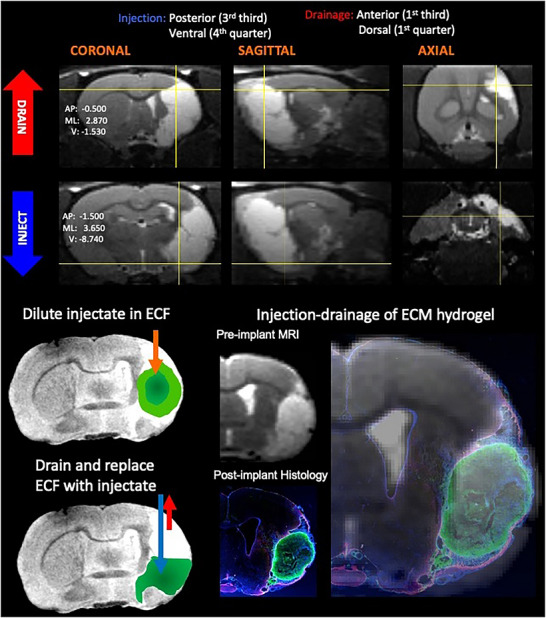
Image‐guided bioscaffold delivery. Magnetic resonance imaging (MRI) is used to measure the cavity volume, as well as to define stereotactic coordinates for the positioning of injection and drainage cannulae.^[^
